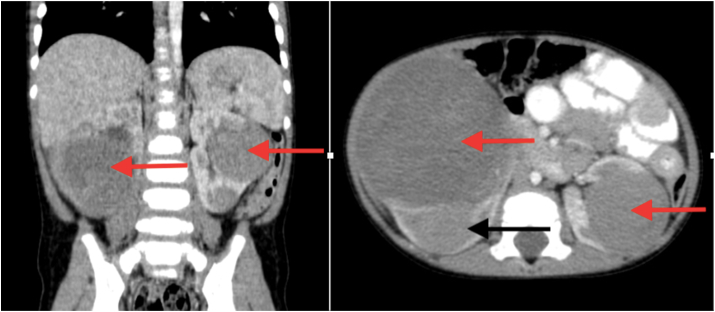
Axial CT image from a patient with Wilms tumor showing the characteristic “claw sign” seen in tumors of renal origin, in which the enhancing renal parenchyma is displaced by the enlarging tumor.
